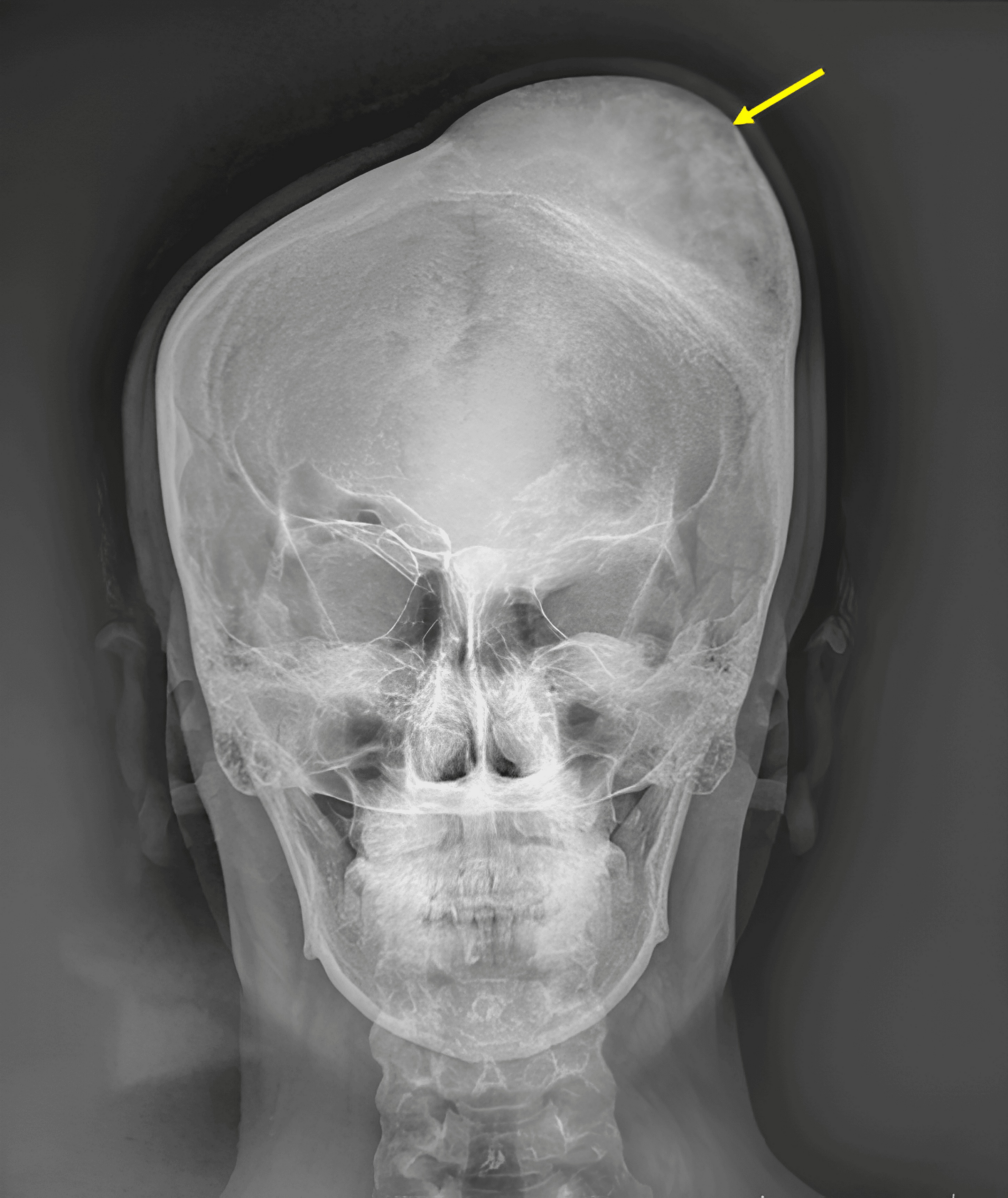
Plain skull X-ray revealing a significant deformation of the vault in the left frontoparietal region (arrow).
